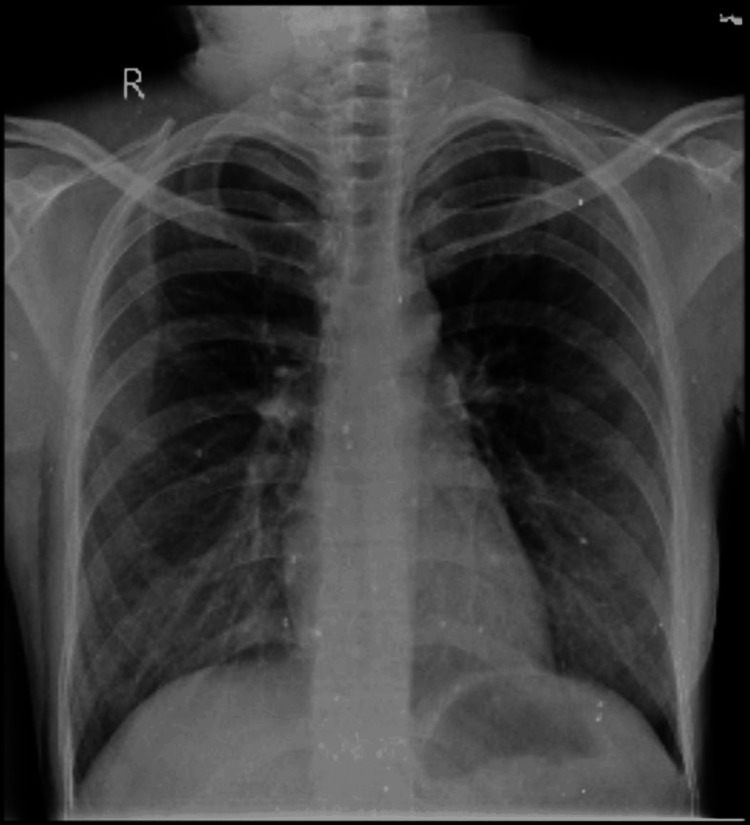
Chest X-ray Interpretation: heterogeneous opacity in the B/L lower and middle zones; prominent bronchovascular marking present in the middle and lower zones
